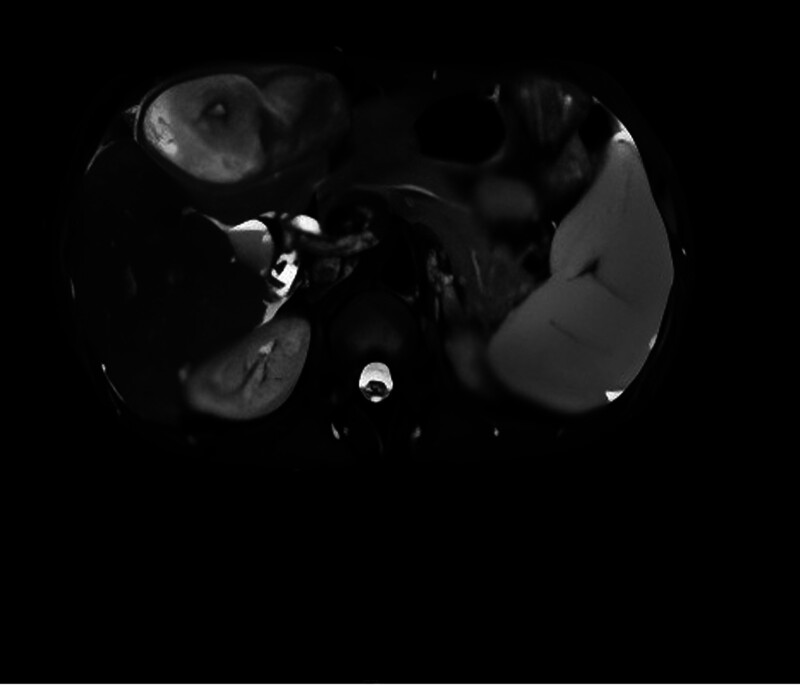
Epigastric MRI plain + enhanced + MRCP + DWI: malignant mass with hemorrhagic necrosis in left lobe of liver, compensatory enlargement of right lobe of liver, unclear display of hepatic veins, hepatic segment stenosis of inferior vena cava, consistent with Budd-Chiari syndrome. DWI = diffusion weighted imaging, MRCP = magnetic resonance cholangiopancreatography, MRI = magnetic resonance imaging.
